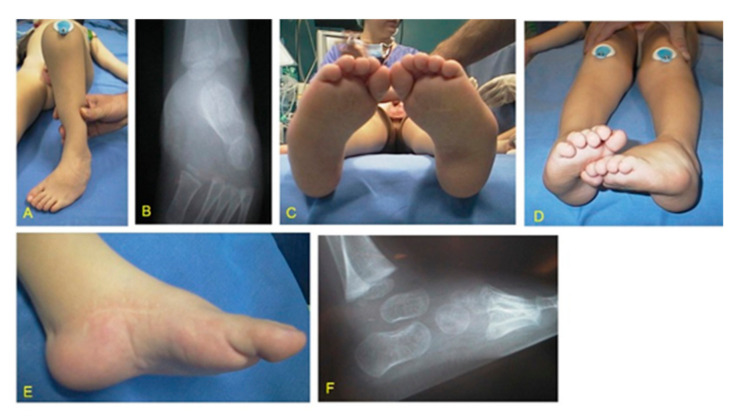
MS (Moebius syndrome) patient with bilateral stiff CF (clubfoot) at 3.5 years of age at the time of relapse surgery. ATL (Achilles tendon lengthening), PR (posterior release), and MR (medial release) were performed at 5 months of age. (A) Adduction deformity left; (B) X-ray in antero-posterior projection showing pathological talus calcaneal angle; (C) plantar view of both feet showing cavus and adduction deformities; (D) clinical aspect in frontal plane showing all components of CF; (E) medial aspect with skin scars after ATL, PR, and MR; (F) X-ray in lateral projection showing pathological talus calcaneal angle.
